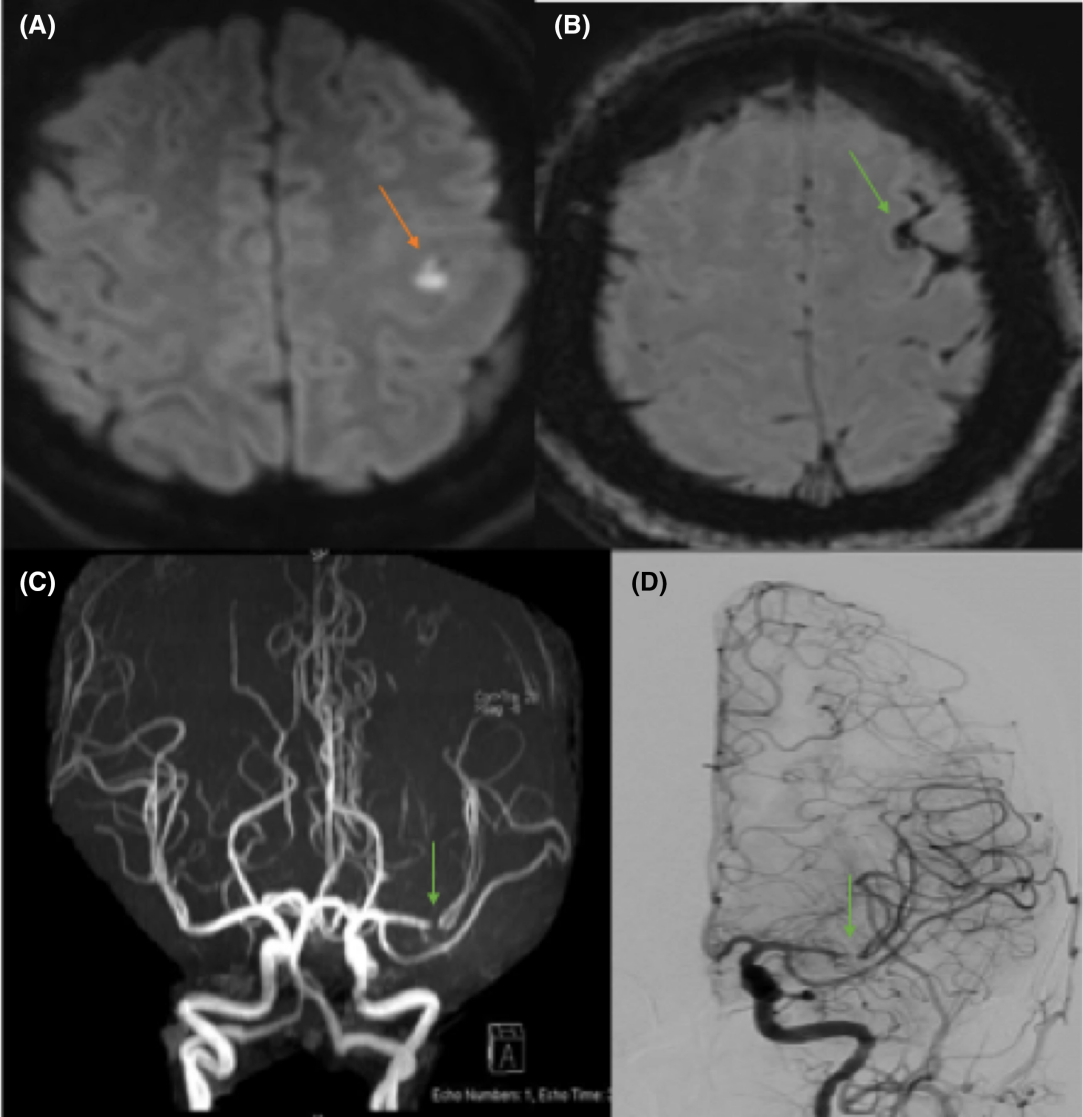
[A–D] Case two with left M1 stenosis an ipsilateral cSAH [A, B] There are effaced sulci on T1, with sulcal high FLAIR signal intensity, and blooming artifact seen within the left frontal sulci corresponding to the high density representing subarachnoid hemorrhage. [C] Cranial MRA: There is focal segment of severe stenosis seen at the M1/M2 of the left MCA, with faint visualization of the proximal M2 branches and paucity of distal M3 branches [D] Cerebral angiography: Tight stenosis of the distal Lt M1 segment. Sluggish flow in the Lt MCA branches, Lt transverse, and Lt sigmoid sinuses
